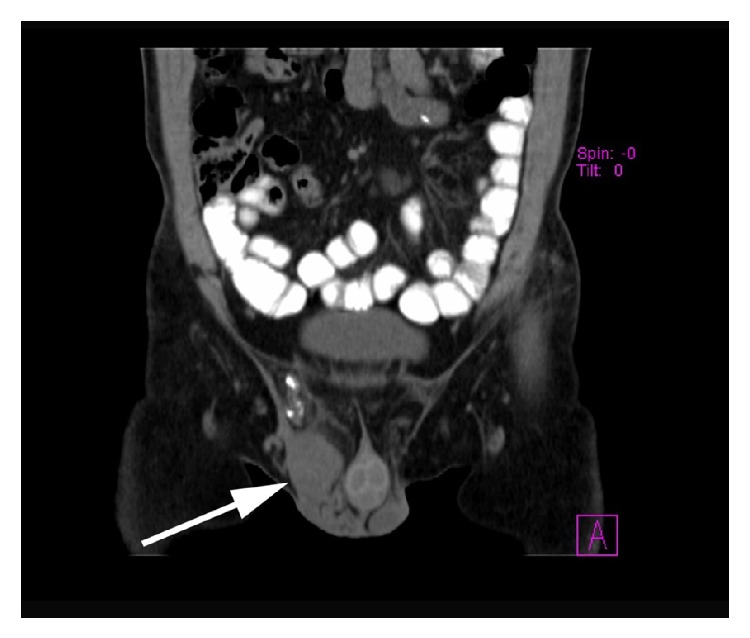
CT scan showing a solid soft-tissue mass of 3.6 × 3.7 × 5.8 cm in the right scrotum (white arrow).
